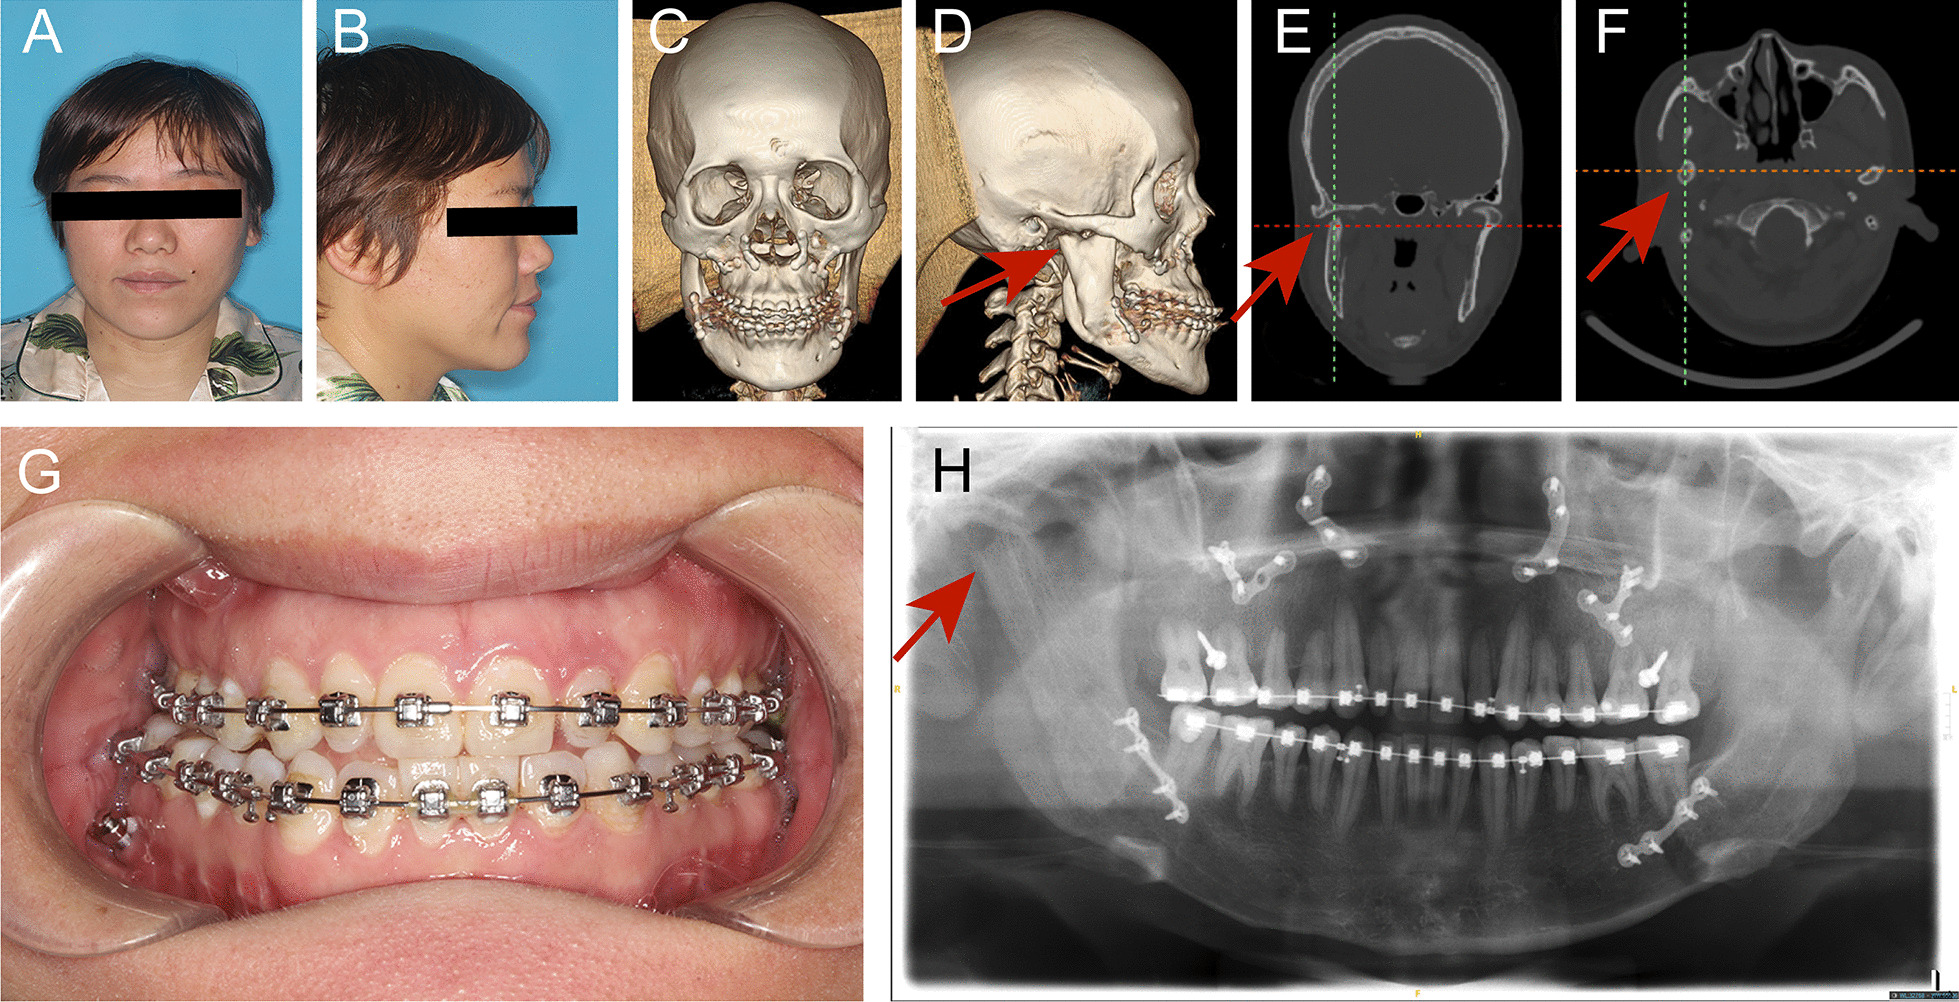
Data collection at 1 year after surgery. a front profile; b lateral profile; c front three-dimensional CT image; d lateral three-dimensional CT image; e coronal CT scan; f horizontal CT scan; g intraoral view; h panoramic radiograph. Red arrow, neocondyle
